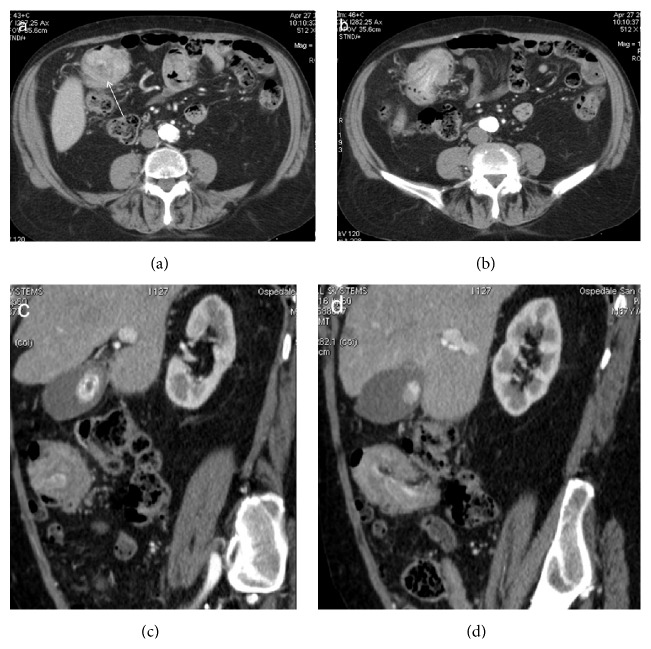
Enterocolonic (ileocaecocolonic) intussusception caused by a polyp. Axial CT images (a, b) demonstrate a soft-tissue density round mass with thin eccentric hypodensity (arrow). Oblique CT reformatting (c, d) clearly shows the enhancement of the lead mass, which facilitates its identification.
